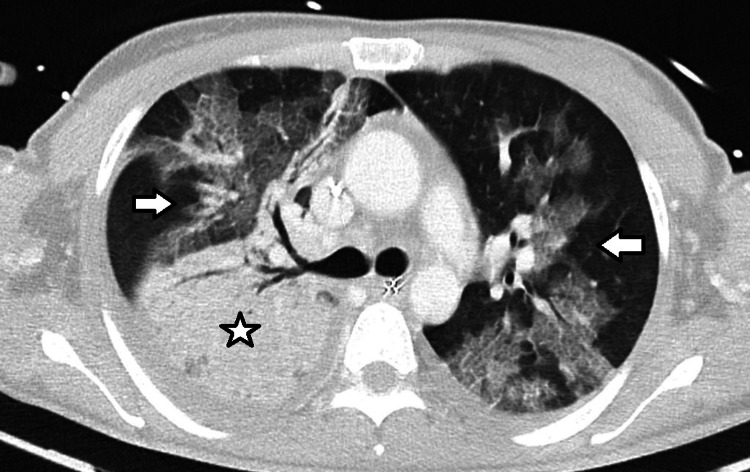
First CT scan showing extensive areas of consolidation (star) and ground-glass opacities (arrows), more pronounced in the right upper lobe, with some associated septal thickening. CT, Computed tomography
